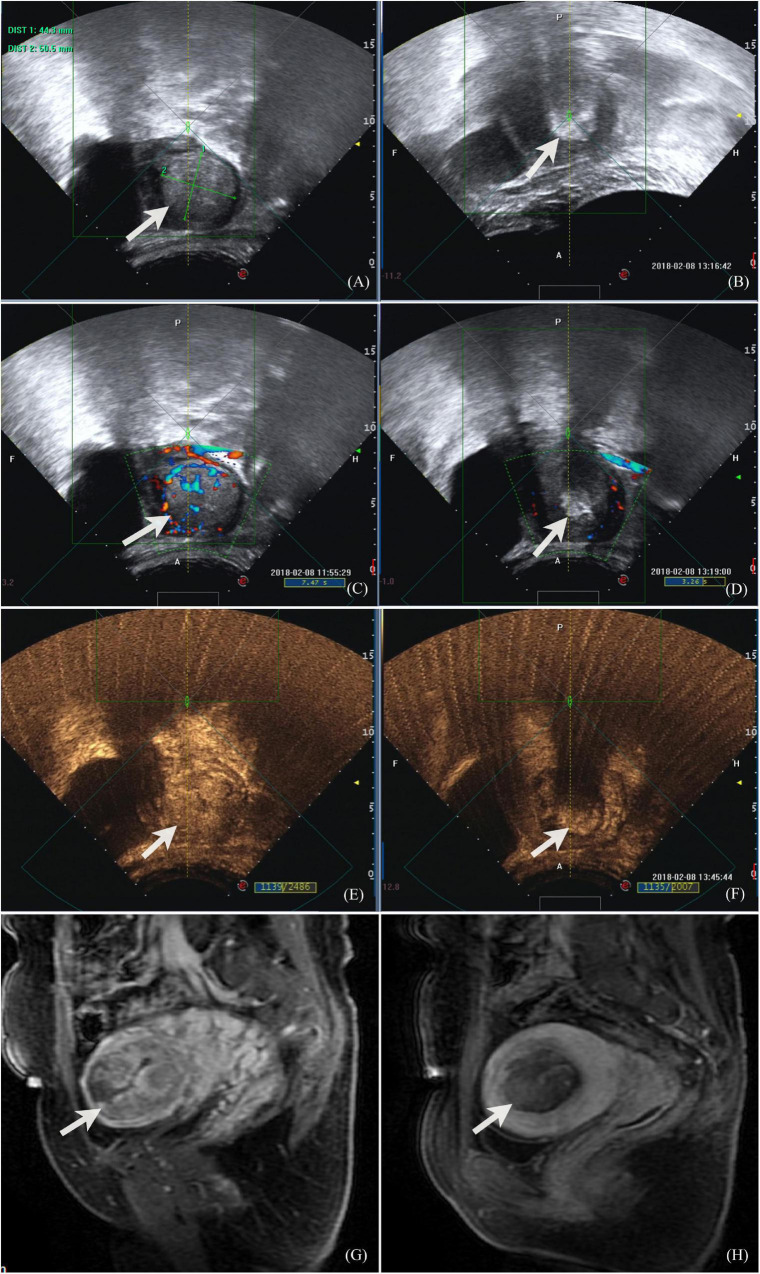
Ultrasound and MR images of a 32-year-old patient with placenta increta. (A) Before HIFU ablation, monitoring ultrasound showed placenta invasion on the fundus-posterior wall of the uterus, about 50.5 × 44.3 mm, with thin myometrium surrounding; (B) during the procedure of HIFU ablation, the monitoring images showed that the target area was clumped with enhanced echo; (C) color Doppler flow imaging showed abundant blood flow signals both in the lesion and surrounding before HIFU ablation; (D) color Doppler flow imaging showed that the grayscale increased in the lesion and no blood flow signal was observed, also peripheral blood flow signal decreased and disappeared after HIFU ablation; (E) contrast-enhanced ultrasound showed that there was abundant blood perfusion in the lesion before HIFU ablation, and there were lacunar areas with less blood flow; (F) contrast-enhanced ultrasound showed that after HIFU ablation, the lesions were covered by grayscale increased images, and the posterior acoustic attenuation was observed. There was no blood perfusion in the lesions, and the boundary between the lesions and the surrounding uterine myometrium was clear; (G) contrast enhanced MR image showed that abnormal signal lesion enhanced in the intrauterine before HIFU and thin myometrium in the fundus of uterus; (H) contrast enhanced MR image 1 day after HIFU showed that the lesion in the uterine cavity was ablated with no blood perfusion.
